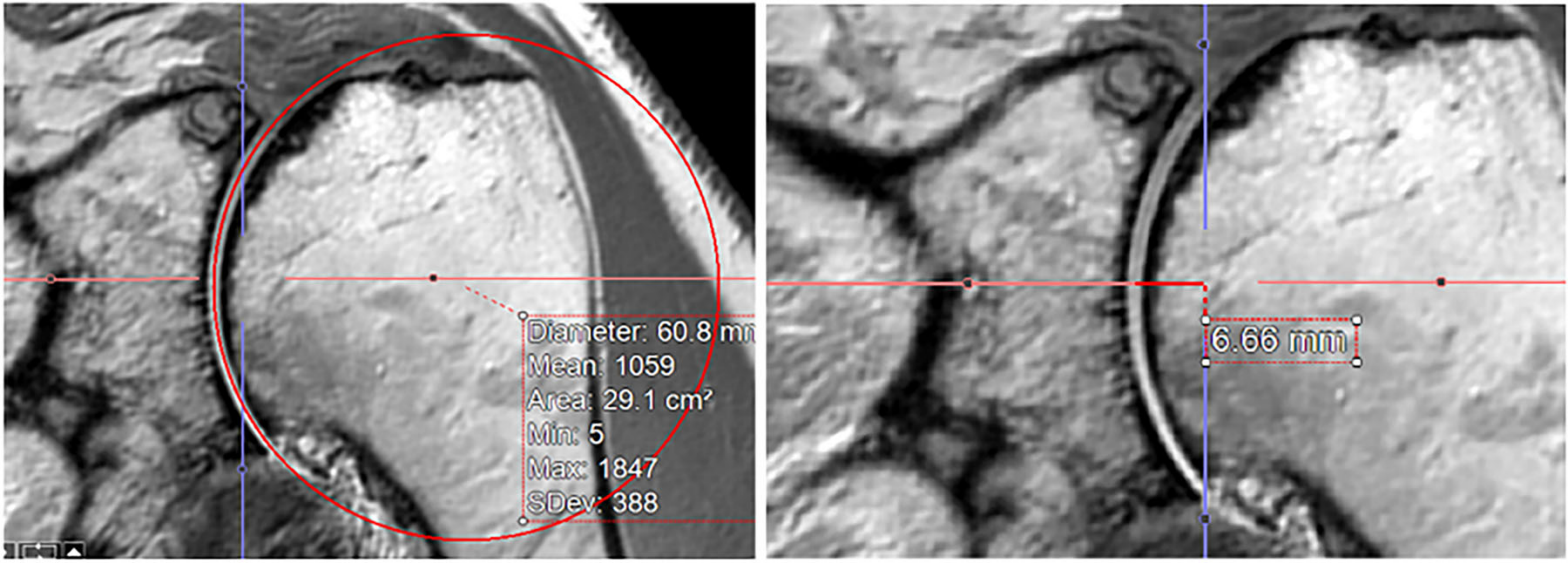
Example of radius and depth measurement in magnetic resonance imaging, considering cartilage and labrum of a left glenoid in superior‐inferior direction. The radius on the left picture was measured using a best‐fit circle, matching the curvature of the glenoid without penetrating the articular surface. The glenoid depth on the right picture was measured by aligning a tangent between the most superior and inferior points of the glenoid rim.
